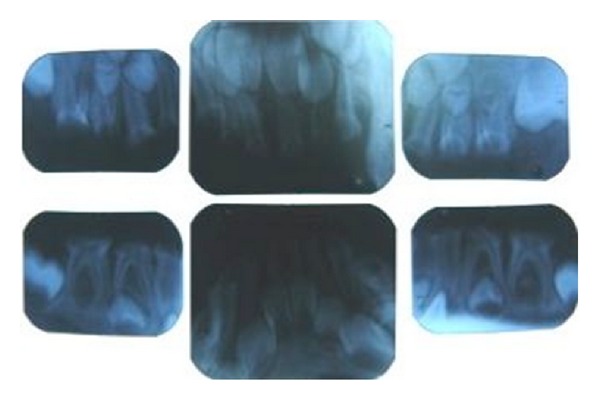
Periapical radiographs showing sEEC.
